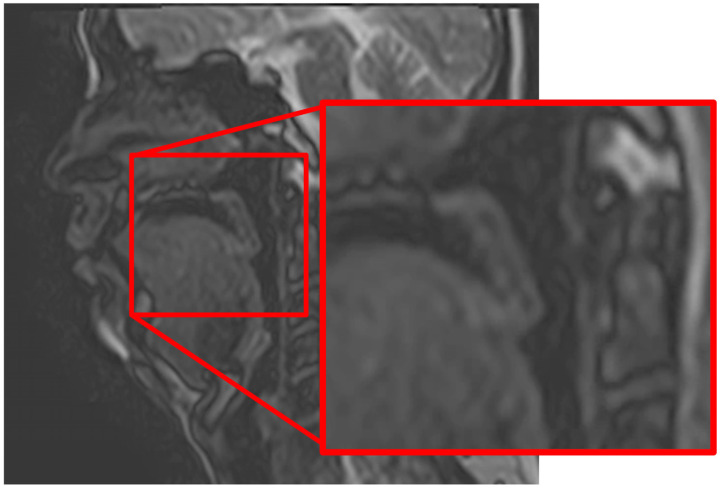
Cine-MR images (TR 3.2 ms, TE 1.6 ms, flip angle 45°, FOV 250 × 225 cm, slice thickness 8 mm, matrix 120 × 96) showing muscle movement and signal changes during articulation of “papa”. During articulation, the associated muscles move in coordination, accompanied by an increase in the signal intensity of each muscle. Supplementary Material Permission has been obtained to publish all figures.
